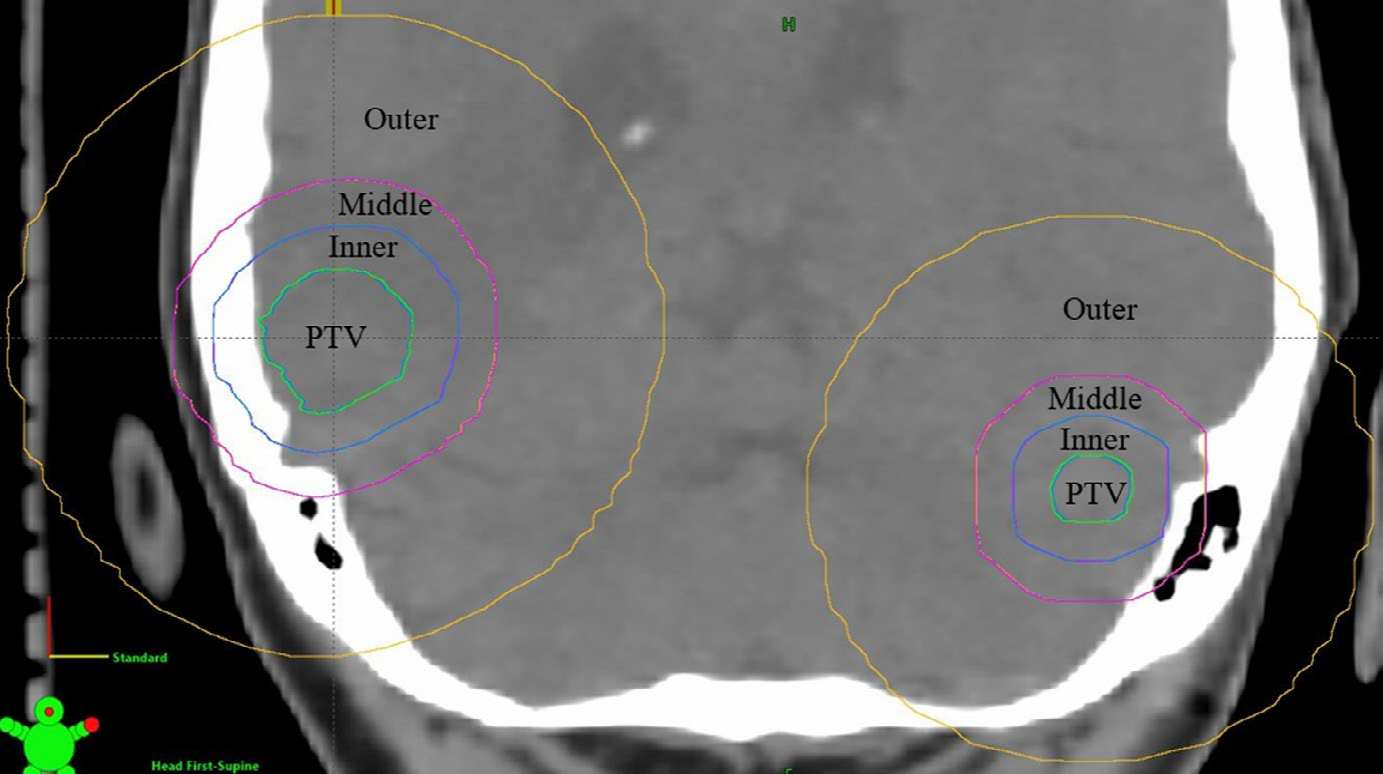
Transverse cross‐sectional CT image of a patient head showing ring‐shaped control regions utilized in the optimization process
